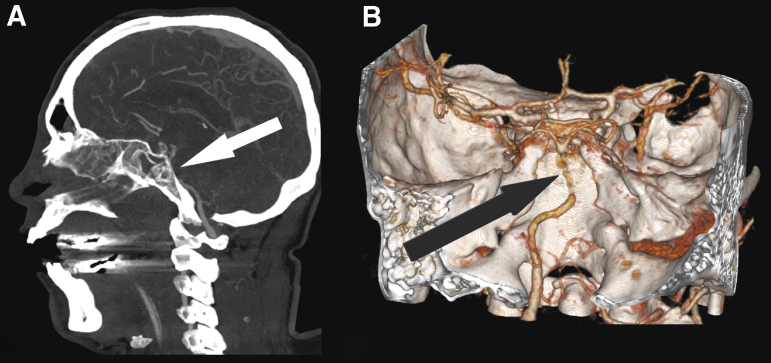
Computed tomography images. (A) Sagittal maximized intensity projection (MIP) image showing basilar artery herniation into the clivus fracture and its trans-sinussoidal course. (B) Three-dimensional (3D) reconstruction of the skull base and the circle of Willis' region displaying the basilar artery entrapment
